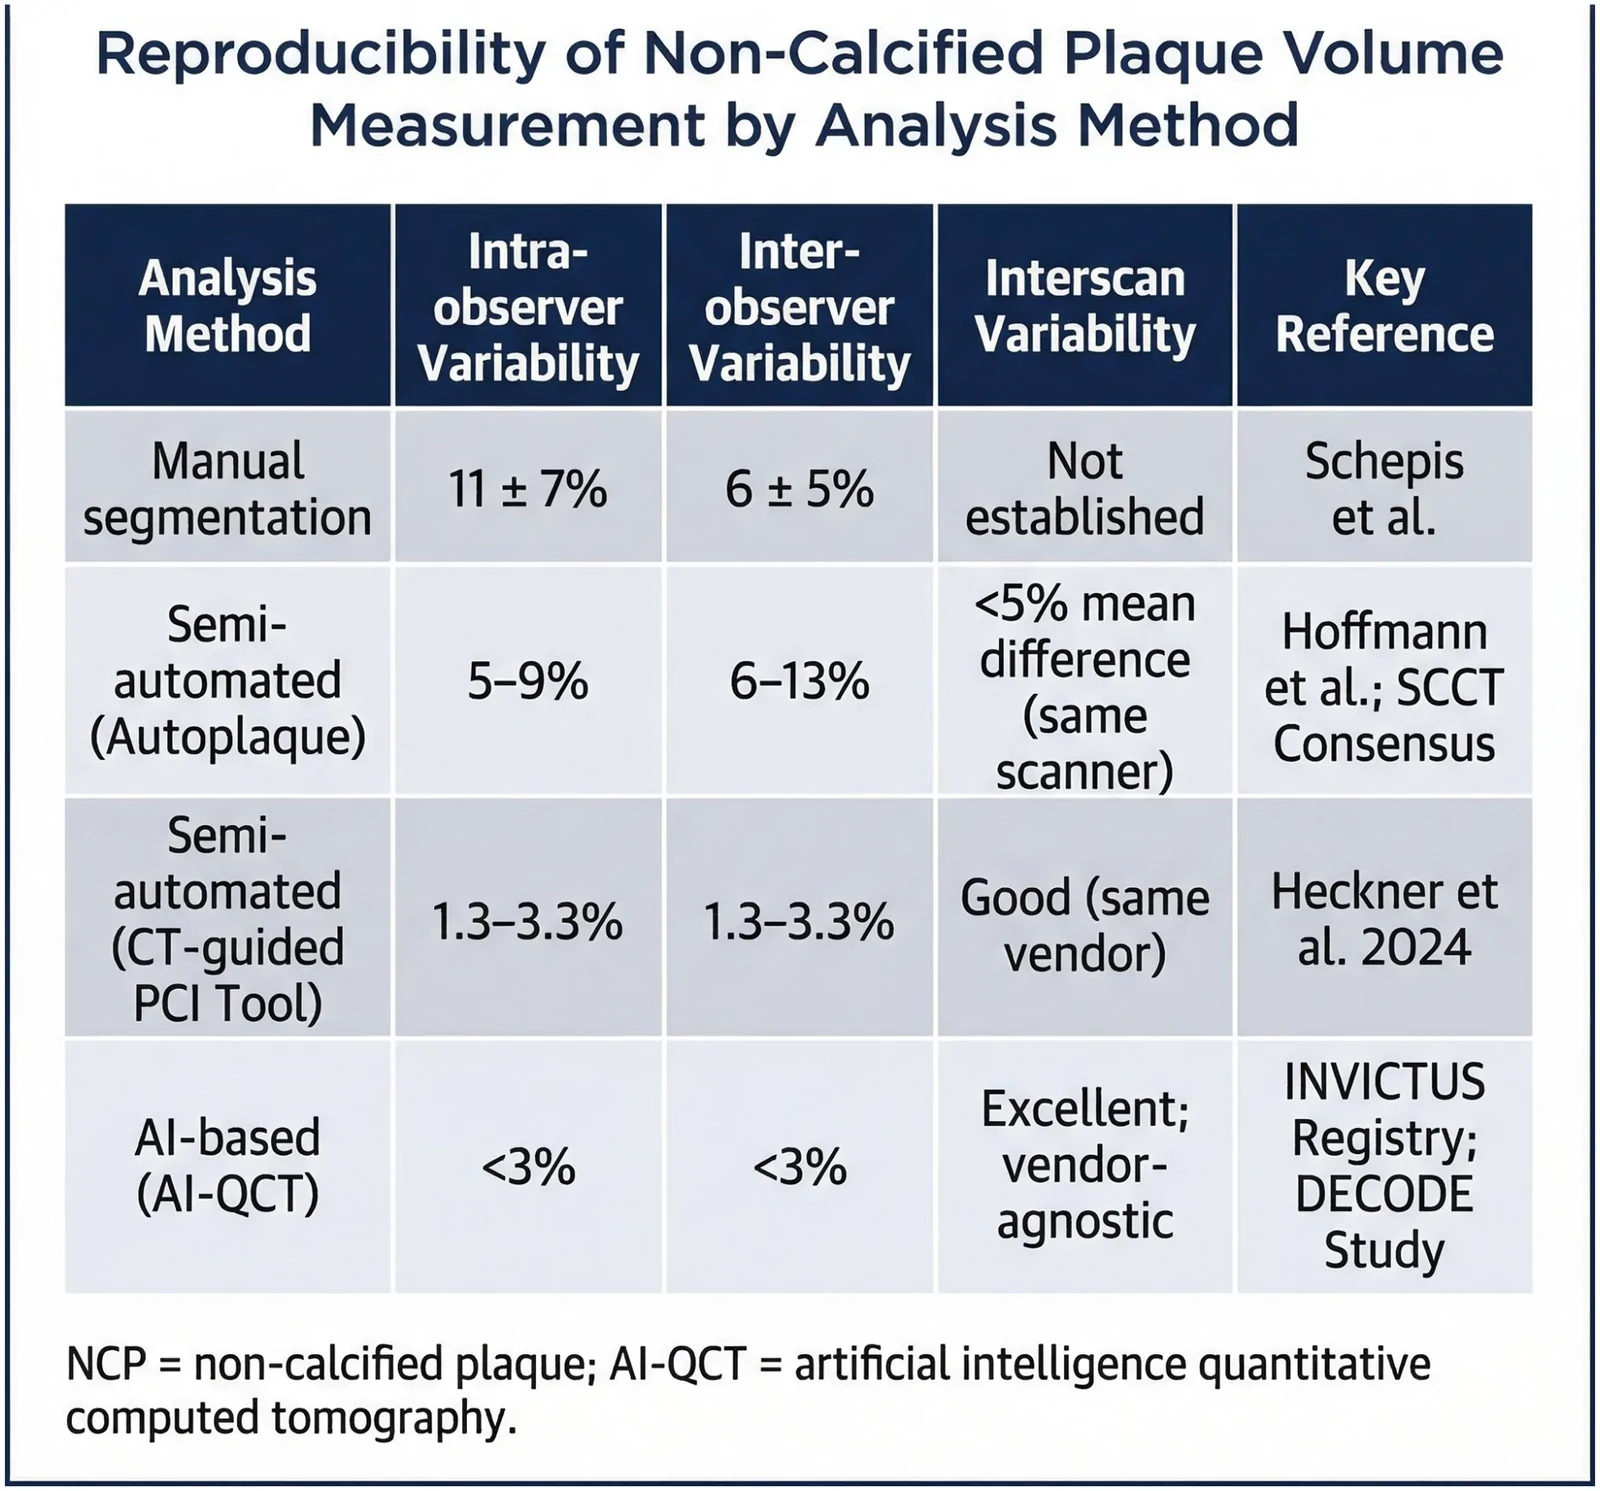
Summary table of reproducibility metrics for non-calcified plaque volume measurement by analysis method. Automated AI-based platforms demonstrate the lowest inter- and intra-observer variability with vendor-agnostic interscan reproducibility. NCP, non-calcified plaque; AI-QCT, artificial intelligence quantitative computed tomography.
